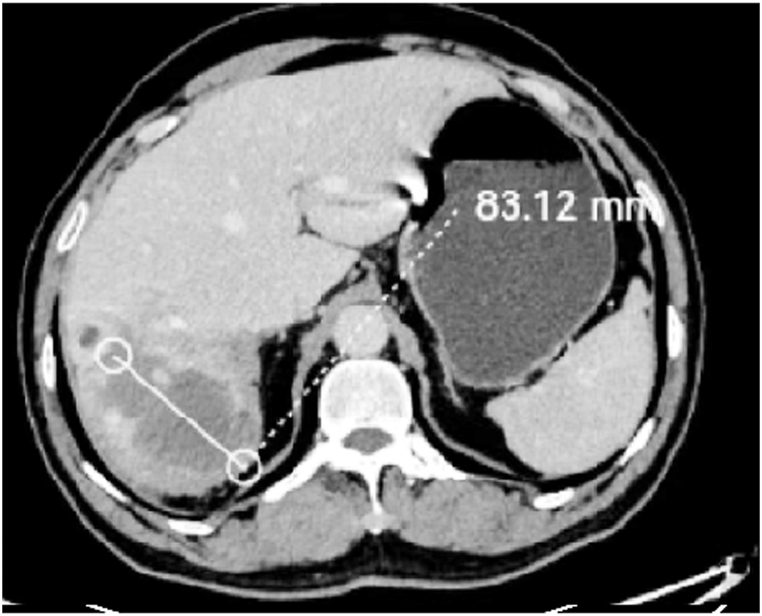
CT image obtained 4.6 months after the first DEB-TACE revealed considerable reduction in CHL size to 83.1 × 50.1 mm.
